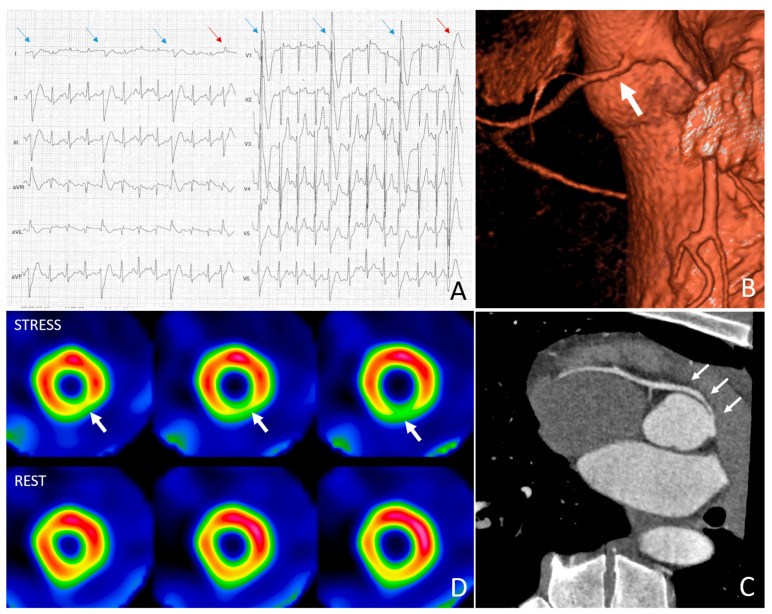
Representative example of an athlete with premature ventricular beats at exercise testing performed for preparticipation screening (A). Further examinations revealed a right coronary artery originating from the left Valsalva sinus with slit-like ostium and narrowing of the interatrial course (B,C). Stress-rest myocardial SPECT revealed reversible ipoperfusion of the infero-lateral left-ventricular wall, tributary to the abnormal coronary artery (D).
